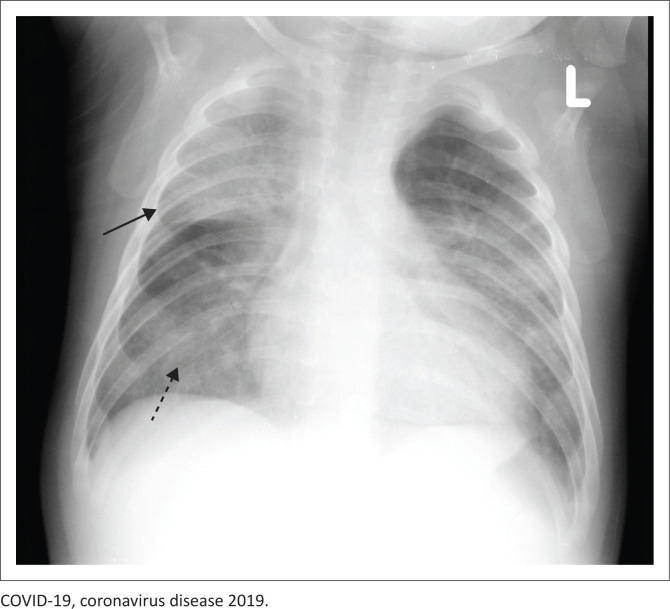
Frontal radiograph of a 4-month-old male patient showing consolidation of the right upper lobe (solid black arrow) and ground-glass opacities (dashed black arrow) in bilateral lung fields. This infant had COVID-19 with a superimposed bacterial infection.
